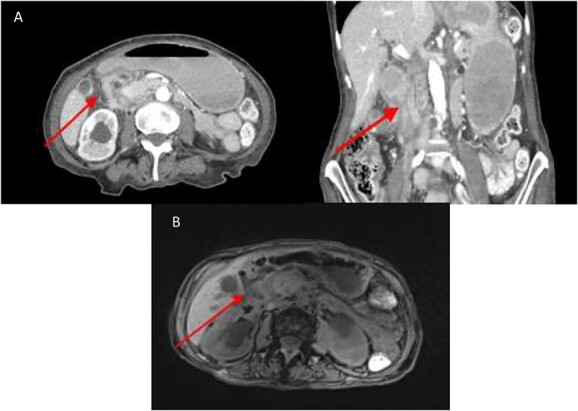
(A) CT abdomen showing marked luminal narrowing (red arrow) at the second part of the duodenum (axial and coronal section). (B). T1 MRI Showing retroperitoneal soft tissue (red arrow) paralleling the second part of duodenum resulting in extrinsic compression.
